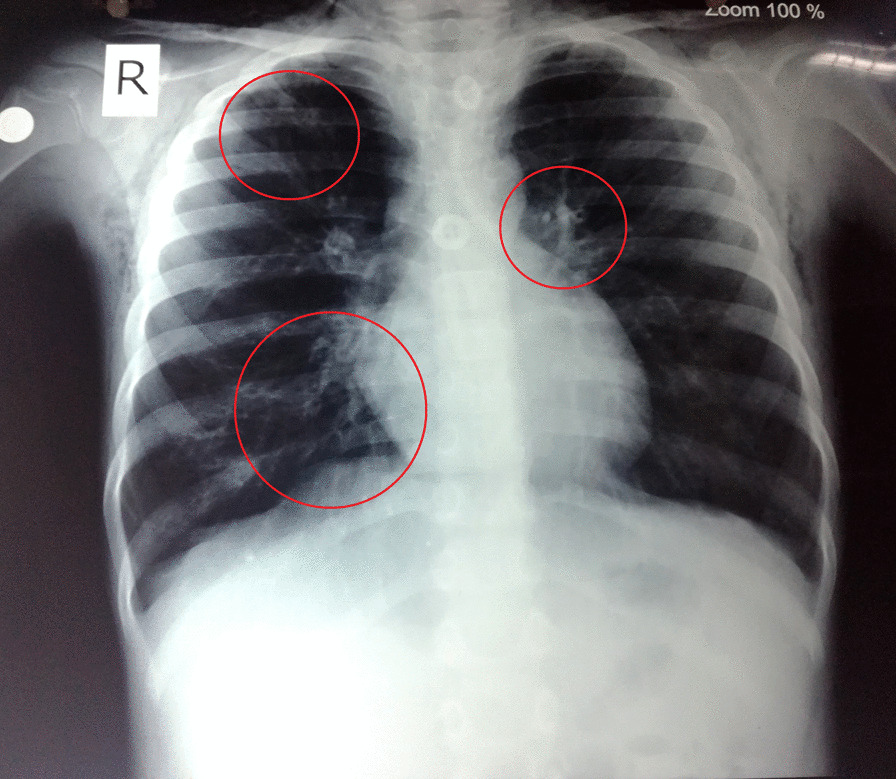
Chest X-Ray showing dilated bronchi in areas circled in red
